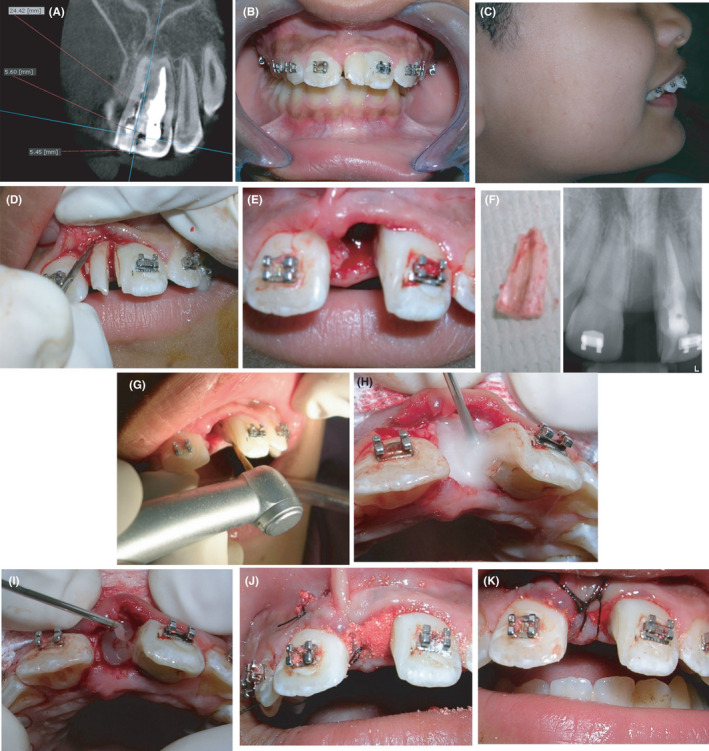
Surgical procedures for sectioning of the fused tooth. (A) Coronal CBCT slice with length measurements to identify surgical plane of sectioning; (B, C) Immediate pre‐operative clinical photos; (D) Use of periotomes for gentle luxation of sectioned supernumerary tooth; (E) socket following removal of supernumerary tooth; (F) extracted sectioned tooth and immediate post‐sectioning periapical radiograph; (G) smoothening of the resected surface; (H) socket treatment with Straumann PREF gel prior to Emdogain^®^ placement; (I) injection of Emdogain® along resected surface and in socket; (J) bioactive glass packed in the socket; (K) socket after grafting and suturing procedures
